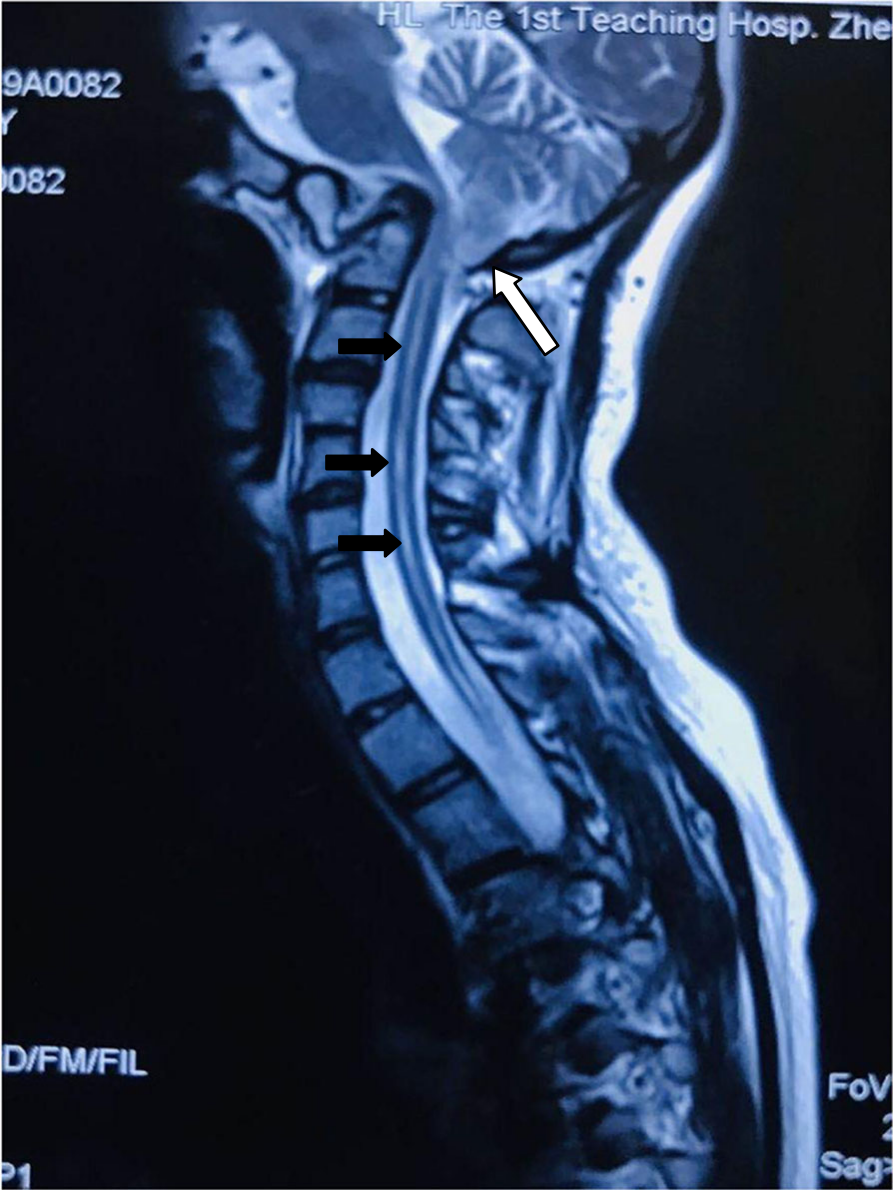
T2-weighted sagittal MRI of the upper spinal cord and brainstem in a pregnant woman with Congenital Atlantoaxial Dislocation and Chiari type I anomaly accompanied by syringomyelia. The syrinx is indicated from C2 to T 9 (black arrows). The cerebellar tonsil herniates through the foramen magnum (white arrow)
